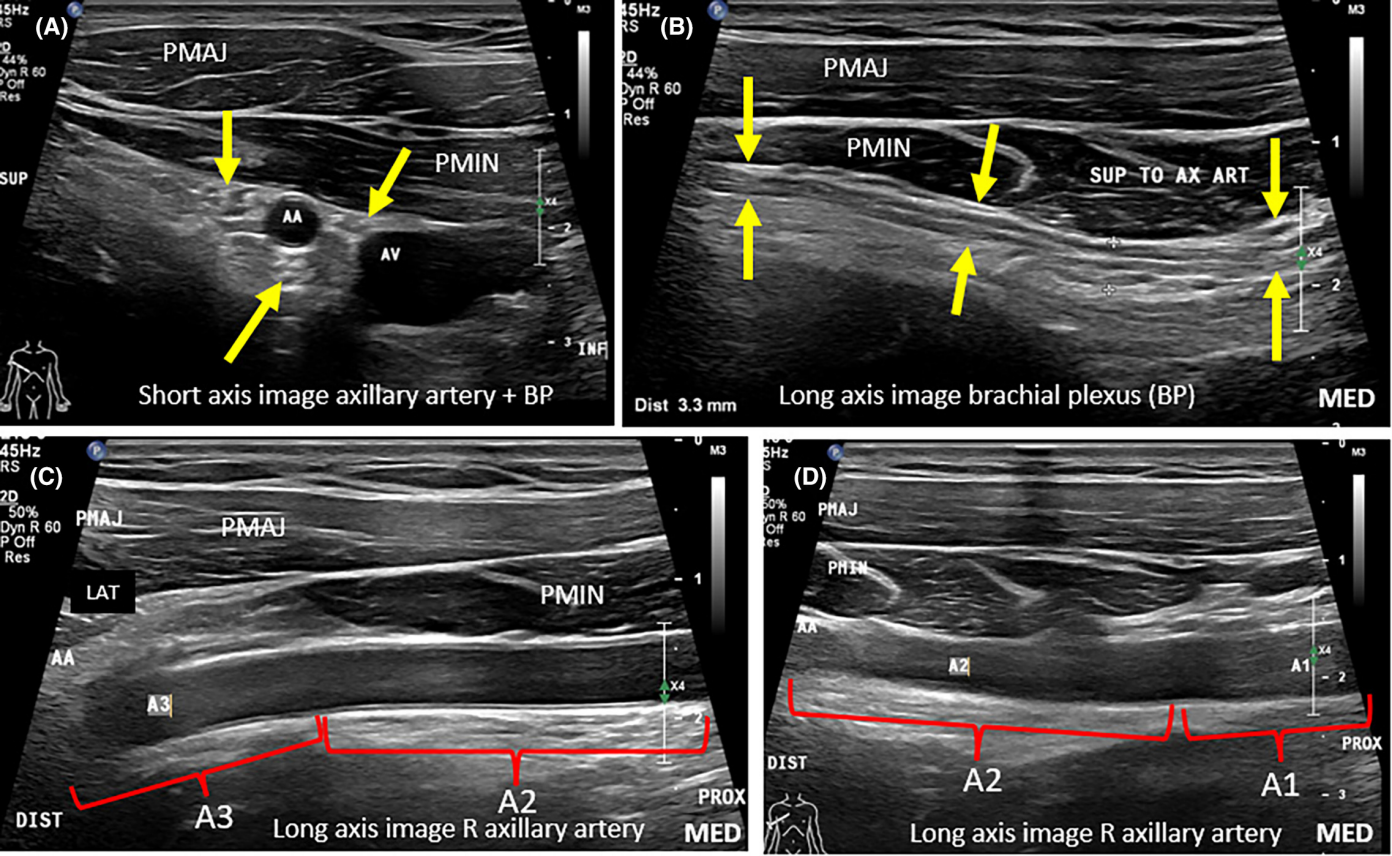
Structures that pass through the axilla. (A). Sonographic image demonstrating the short axis of the axillary artery and brachial plexus (BP) cords (yellow arrows). Transducer position outlined by body marker. (B). Image demonstrating the long axis of one of the brachial plexus cords (yellow arrow). (C). Sonographic image demonstrating the long axis of parts 3 (A3) and 2 (A2) of the right (R) axillary artery. Part 3 is located lateral to the pectoralis minor muscle, and part 2 is located deep to the pectoralis minor muscle. (D). Sonographic imaging demonstrating part 2 (A2) and part 1 (A1) of the axillary artery. AA, axillary artery; AV, axillary vein; MED, medial aspect of long‐axis images; PMAJ, pectoralis major muscle; PMIN, pectoralis minor muscle.
